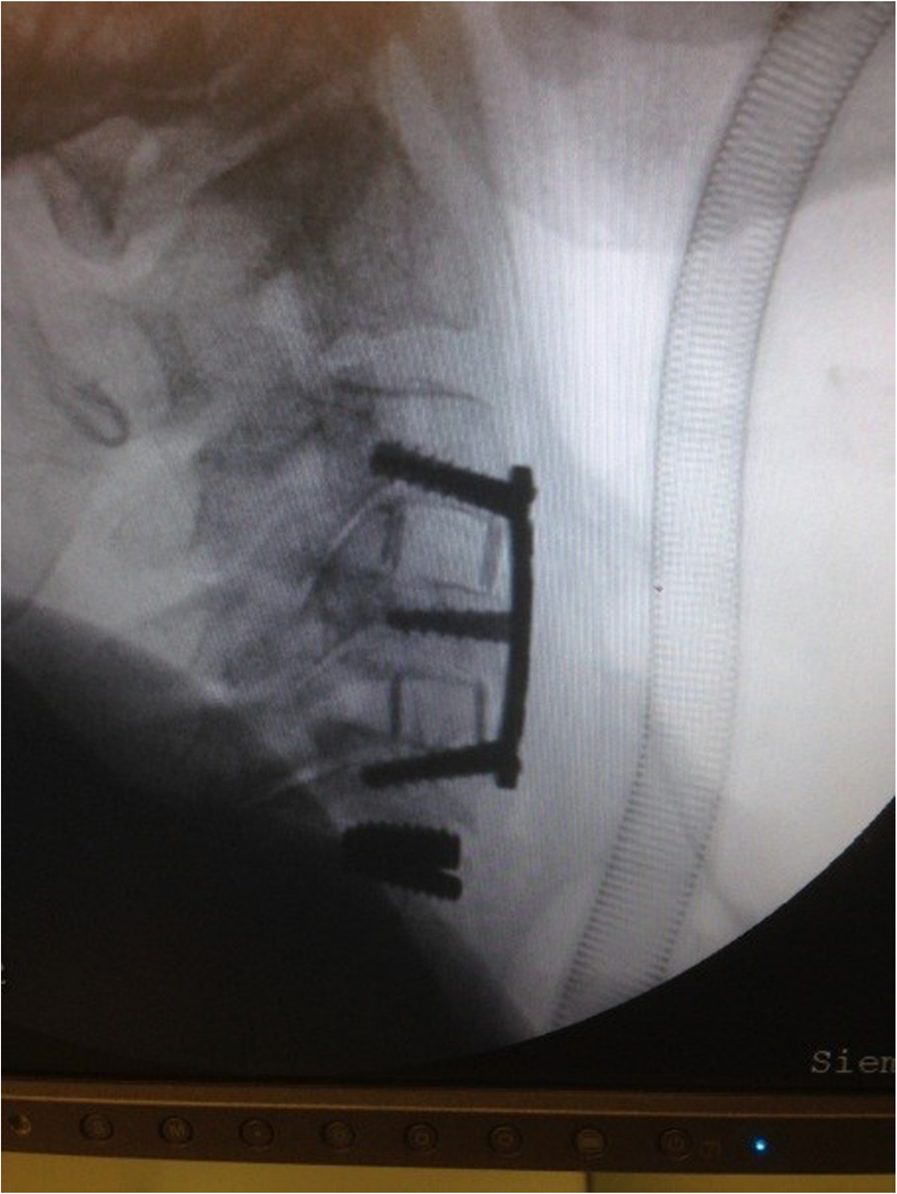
Plain radiograph indicating hybrid construction with anterior fusion for C3–C4 and C5–C6 and cervical disc prosthesis for C6–C7
